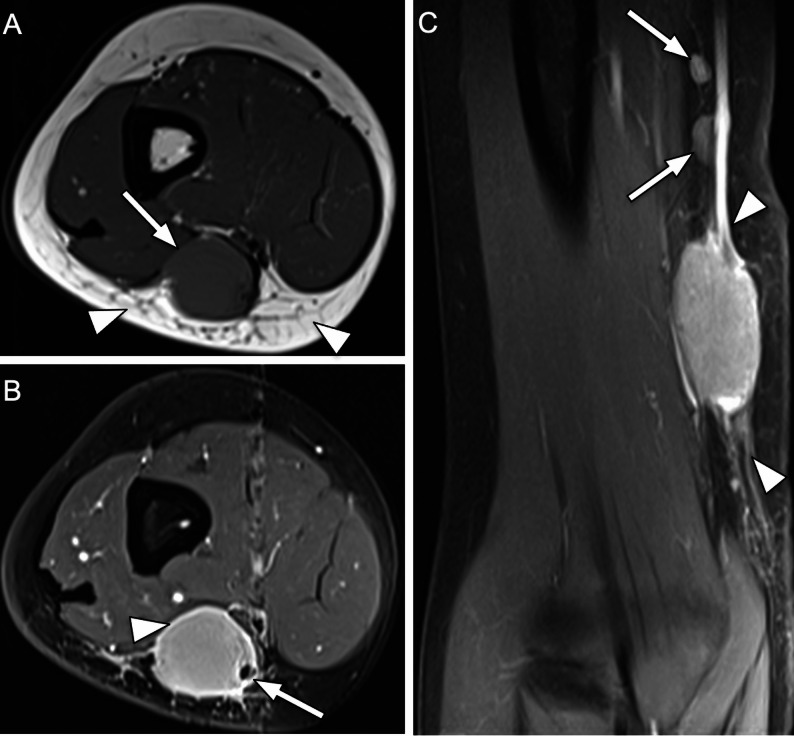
MRI of a 44-year-old female presenting with non-Hodgkin lymphoma manifestation in the upper arm. A Transversal T1-weighted image shows a subcutaneous tumor (arrow), presenting with slightly hyperintense signal intensity compared to muscle and subcutaneous stranding (arrowheads) B Transversal contrast enhanced fat-saturated T1-weighted image shows peripheral enhancement (arrowhead) accompanied by slightly less intensive homogenous enhancement of the remaining tumor. Additionally, encasement of the basilic vein (arrow) is recognizable. C Coronal contrast enhanced fat-saturated T1-weighted image shows growth oriented along the basilic vein (arrowheads) and close association of two additional lesions (arrows) with the basilic vein
